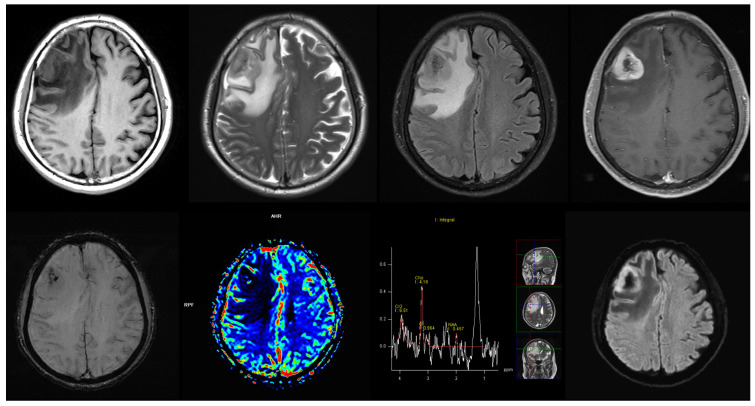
A representative example of multimodal magnetic resonance imaging data from a patient with atypical primary CNS lymphoma. The lesion in the right frontal lobe demonstrated intratumoral necrosis and hemorrhagic components, imaging features that can mimic metastasis or glioblastoma. However, pathology confirmed the diagnosis of lymphoma.
